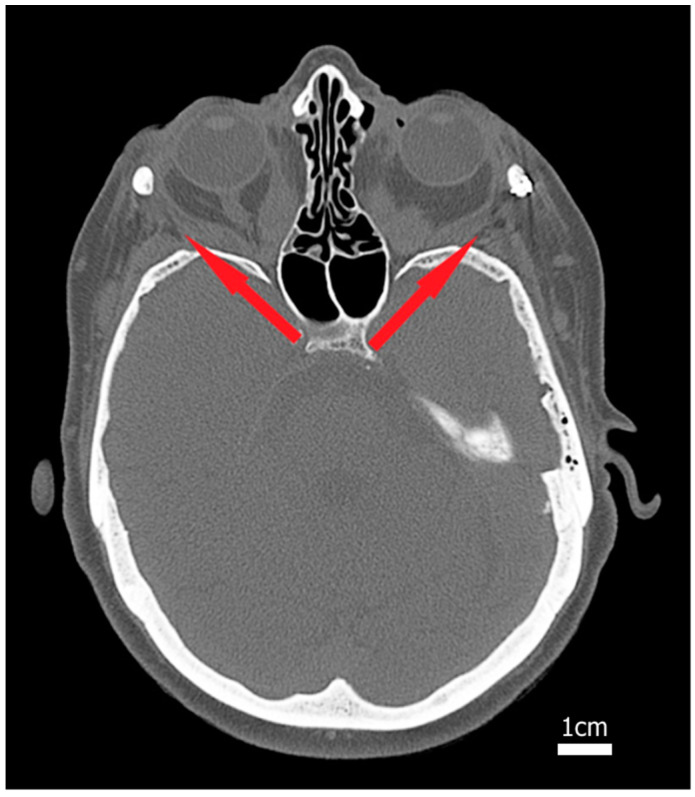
Example of TED treatment using the two-wall resection approach. A central CT slice of a TED patient after two-wall resection treatment (lateral and medial) at both sites. The close relationship of the temporalis muscle to the rectus lateralis muscle can be seen; thus, the laterally open orbit (red arrow) can be closed by the function of the temporalis muscle.
